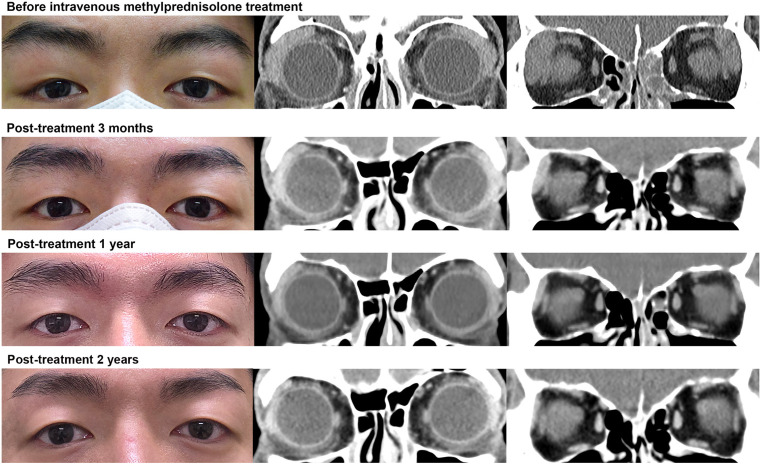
A representative case demonstrating the sustained lacrimal gland volume reduction effect of intravenous methylprednisolone treatment. Face photography and coronal computed tomography images (Top row) before treatment, (Second row) 3 months after treatment, (Third row) one year after treatment, and (Bottom row) 2 years after treatment. Gradual improvement in both upper lid lateral swellings was observed, along with a sustained decrease in lacrimal gland volume. The post-treatment to pre-treatment volume ratio of the left lacrimal gland was 38.4% at 3 months, 30.0% at 6 months, 21.6% at 1 year, and 18.7% at 2 years.
